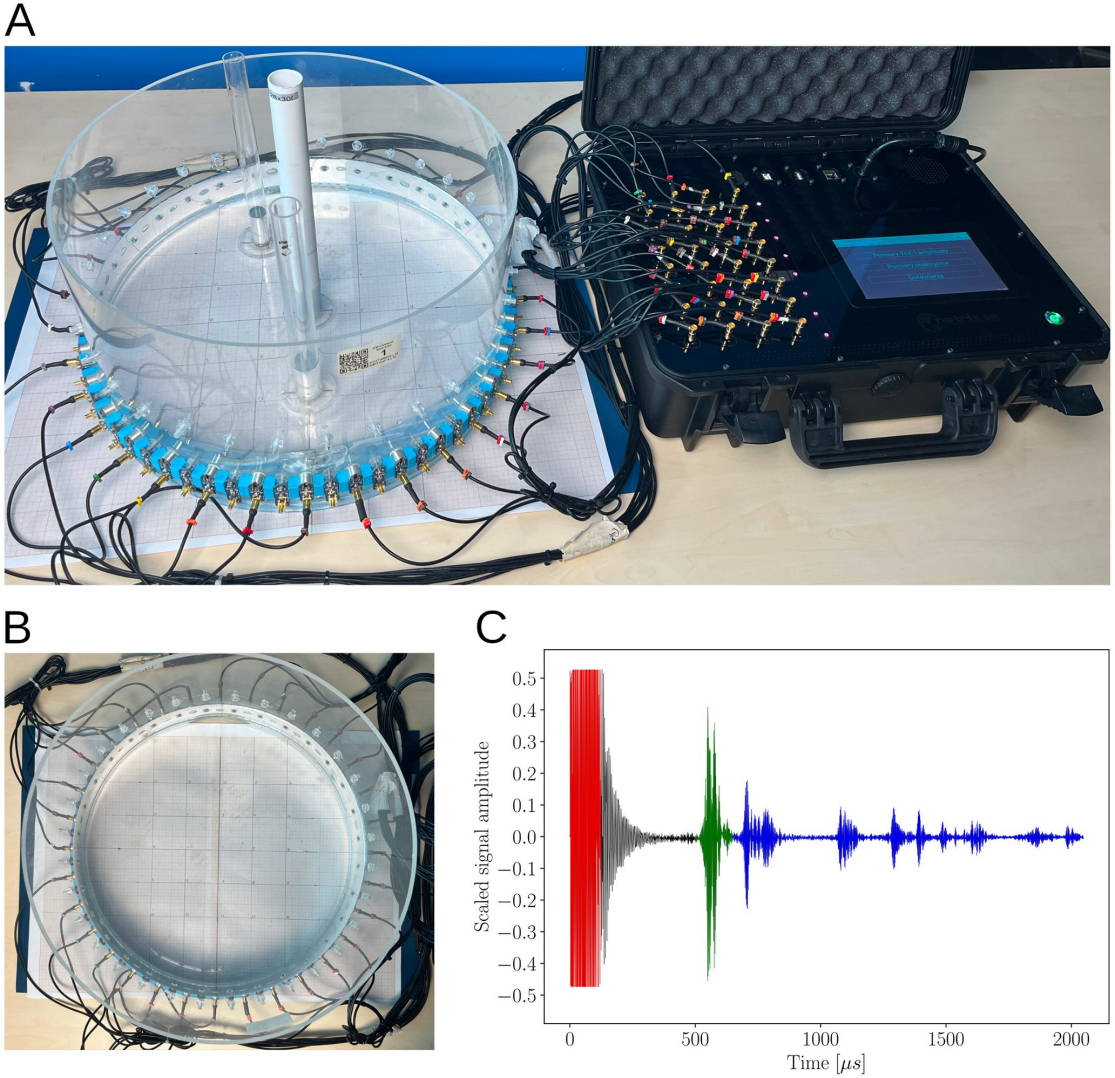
The experimental setup and example of measurement data: a) picture of a complete experimental setup, b) an experimental tank equipped with 32 ultrasound sensors arranged clockwise, c) an example of recorded data from a channel number 1.
